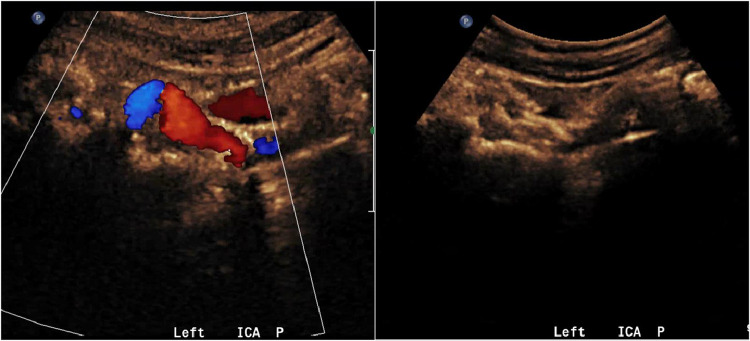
Carotid ultrasound demonstrating calcified plaque formation along the bulb with shadowing.
